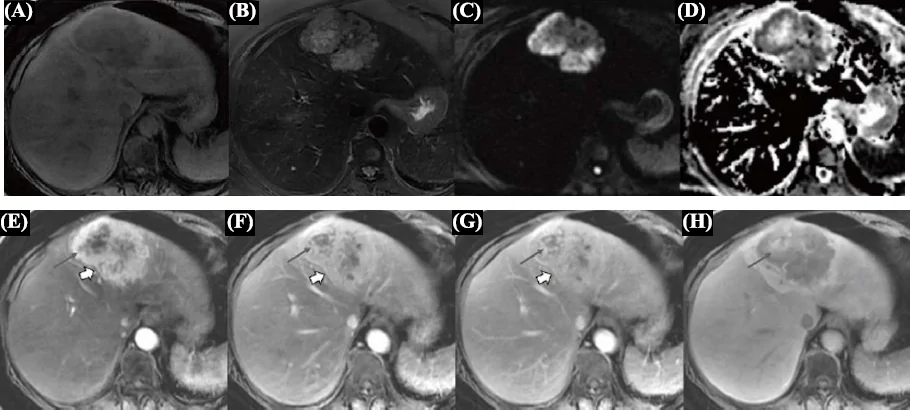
Gd-EOB-DTPA enhanced MRI images.
